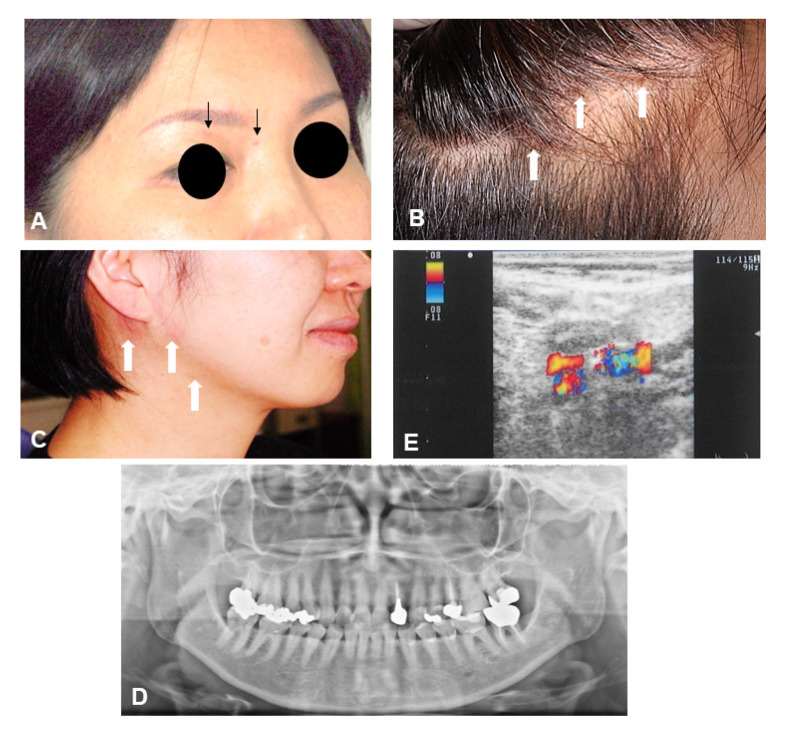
(A–C): The patient had a burning pain, edematous erythema and vesicles, and swelling around the eyelids and on the scalp after a period of days and consulted with a neurosurgeon. (D): Panoramic radiograph shows mild resorption of mandibular condyle on the right side. (E): Ultrasonography showed lymphadenitis.
